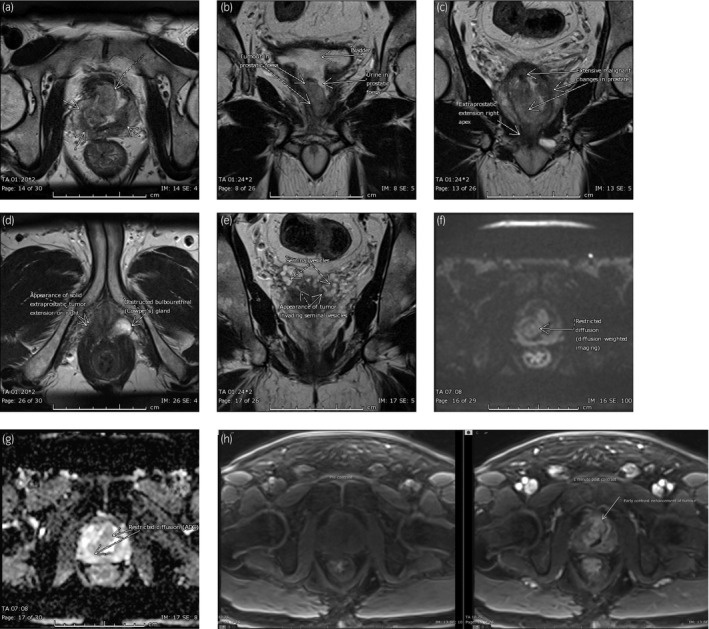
T2 weighted multiparametric MRI showing a bulky and extensive Prostate Imaging‐Reporting and Data System lesion involving the majority of the prostate, centered in the transitional zone and extending to the peripheral zone from base to apex (a, b). Arrows indicate the appearance of solid extra‐capsular extension on the right (c, d) and seminal vesicle involvement (e), not correlated on positron emission tomography/computed tomography. Standard MRI prostate protocol was performed at 3T, including axial DWI (b values 50, 800 and 1200) (f), ADC mapping (g) and dynamic post contrast‐enhanced imaging (h). The tumor demonstrated low to intermediate T2 signal with moderate diffusion restriction and early contrast enhancement with washout.
